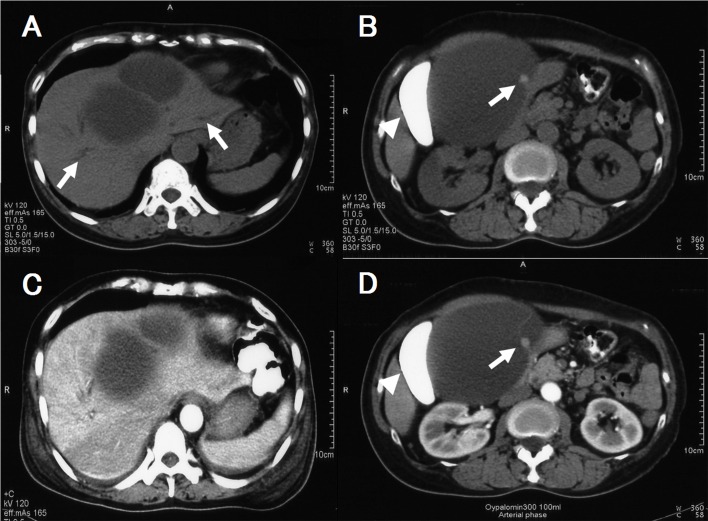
A and B: Plain CT after drip infusion CT cholangiography (DIC-CT), C and D: Enhanced CT after DIC-CT. A and C showed a hepatic cyst with septum near the hepatic hilum and slight dilatation of the intrahepatic duct (arrow). B and D showed a mural module which did not enhance with contrast material (arrow) as well as deformation of the gallbladder (arrowhead).
